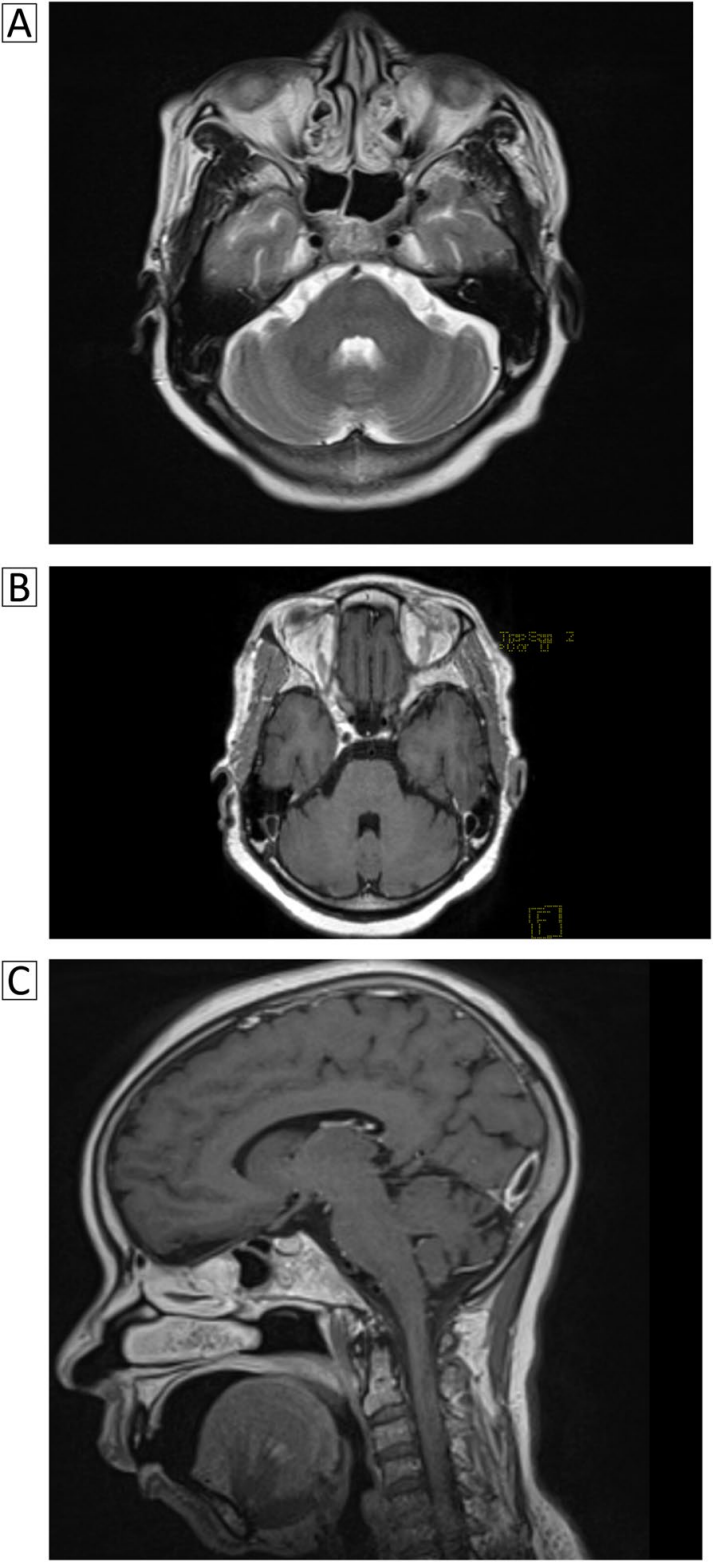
MR imaging findings. MR imaging of the cerebellum and the brainstem did not reveal findings suggestive of rhombencephalitis. A. Axial T2-weighted sequence. B. Axial T1-weighted with gadolinium sequence. C. Sagittal T1-weighted with gadolinium sequence
